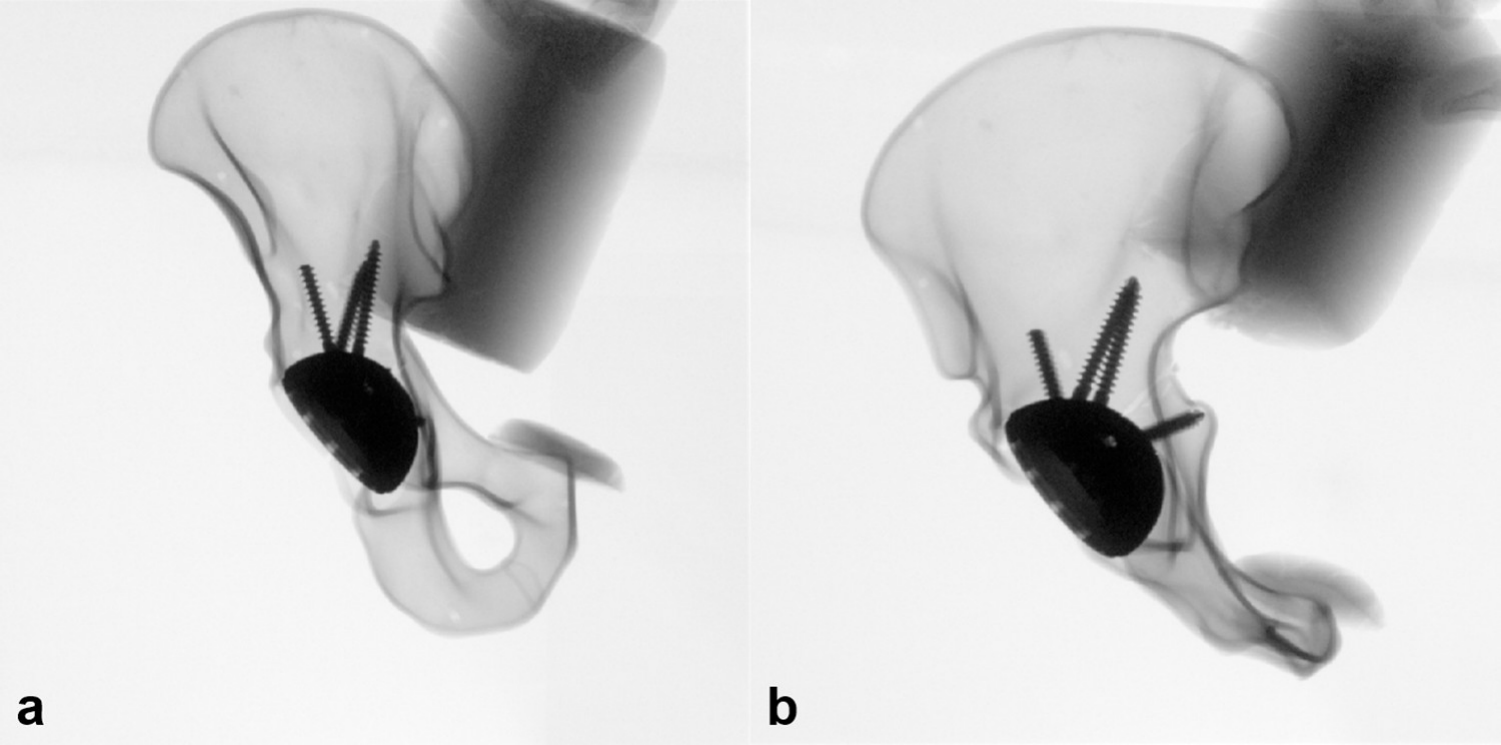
X-ray imaging of the pelvis model with arthroplasty. a shows the a.p.- view and b the ala- view of the hemi-pelvis.
